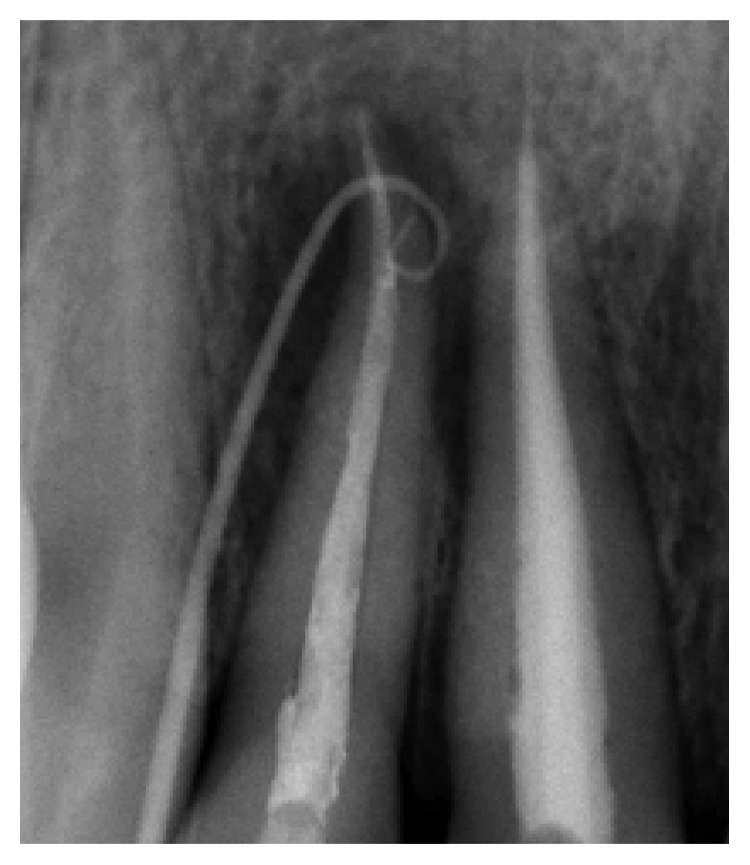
Fistula recurrence after 2 years involving tooth 12, persistence of periapical lesion.
